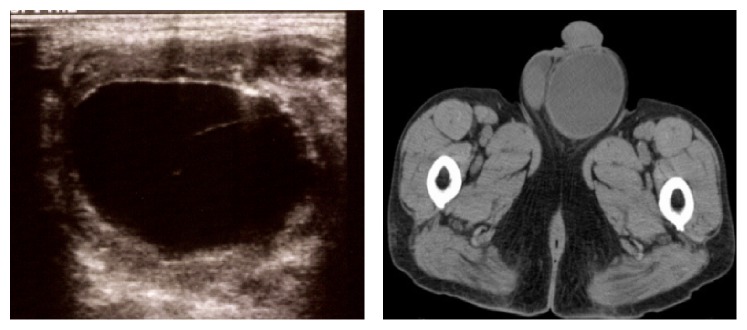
Seroma was diagnosed by ultrasonography (left) or computed tomography (right).
